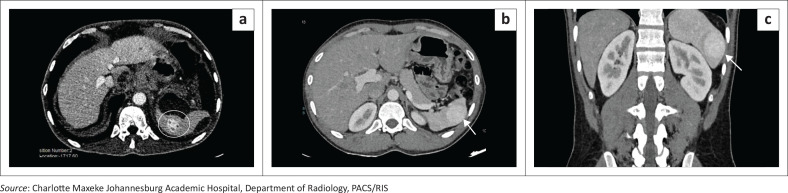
Splenic haemangioma and hamartoma identified incidentally in two patients. (a) Axial contrast-enhanced CT demonstrates a well-defined splenic lesion with characteristic discontinuous peripheral nodular enhancement and progressive centripetal fill-in, consistent with a splenic haemangioma (circle). (b, c) Axial and coronal contrast-enhanced CT images in a different patient demonstrate a well-circumscribed rounded splenic lesion showing relatively homogeneous enhancement (arrows), which may represent either a haemangioma or hamartoma. There is no associated splenomegaly, calcification or surrounding inflammatory change.
